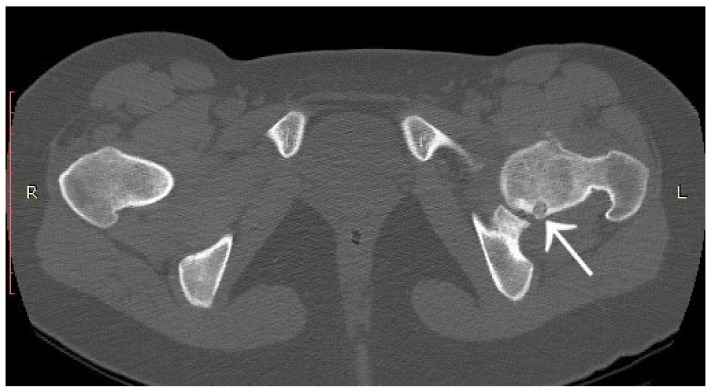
CT scan showing a focus of OO (arrow) in the left femoral head.
